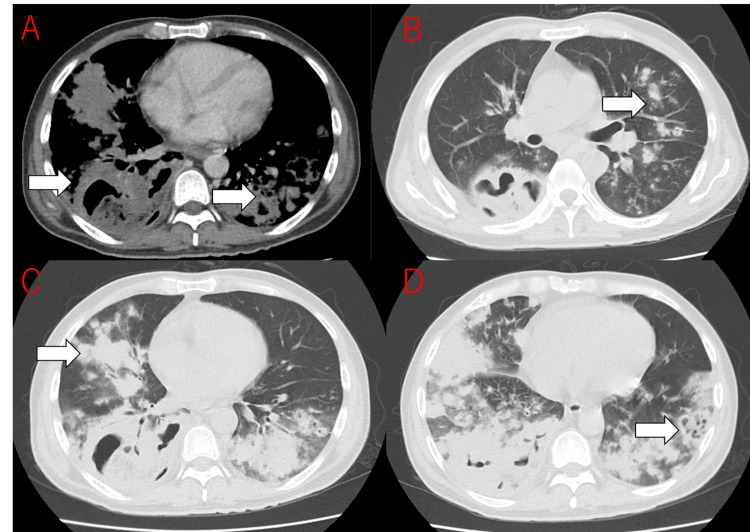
Chest computed tomography scan on admission Axial (A, B, C, D) views demonstrate multiple, bilateral, peripheral nodular infiltrates (arrows) and a distinct cavitary lesion in the lower lobe of the right lung, consistent with septic pulmonary emboli.
